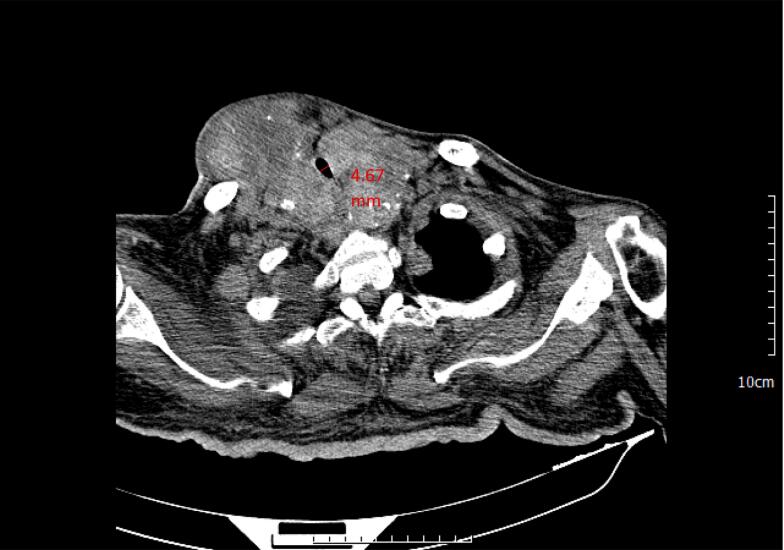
An axial cut of the CT image of the patient's neck showed the presence of a large thyroid mass lesion with heterogeneous density, causing significant asymmetry that displaced adjacent anatomical structures, including vascular and airway components (tracheal internal diameter of 4.67 mm).
